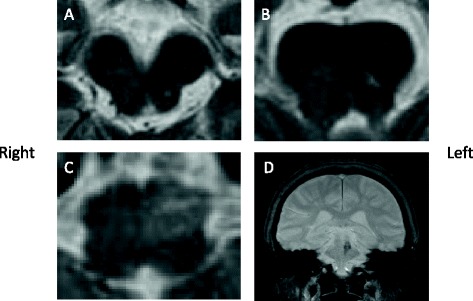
Magnetic resonance images 11 months after the onset of hemorrhage. The hemorrhagic focus in the left tegmentum (A, B, T2-weighted images). High signal intensity in the left medulla oblongata indicating hypertrophic olivary degeneration (C, T2-weighted image). Hemosiderin ring around the lesion in the left pontine tegmentum (D, coronal view, Fast Field Echo-weighted image).
